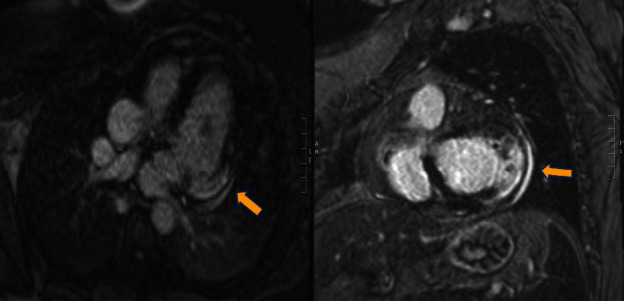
Cardiac magnetic resonance imaging (MRI) showing transmural late gadolinium enhancement in the basal lateral wall (yellow arrows) which is highly concerning of Chagas cardiomyopathy.
